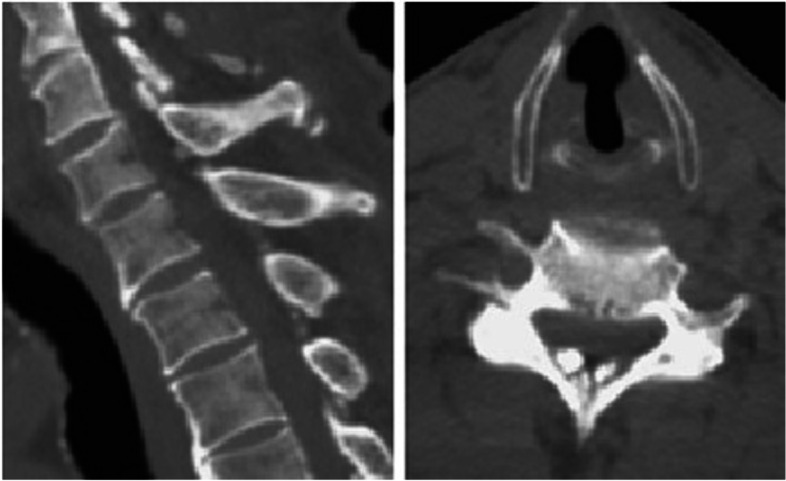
Cervical computed tomography of case 5. Compression of the spinal cord by two main calcified masses was observed on sagittal and axial sections. There is no calcification or ossification of the posterior longitudinal ligament
